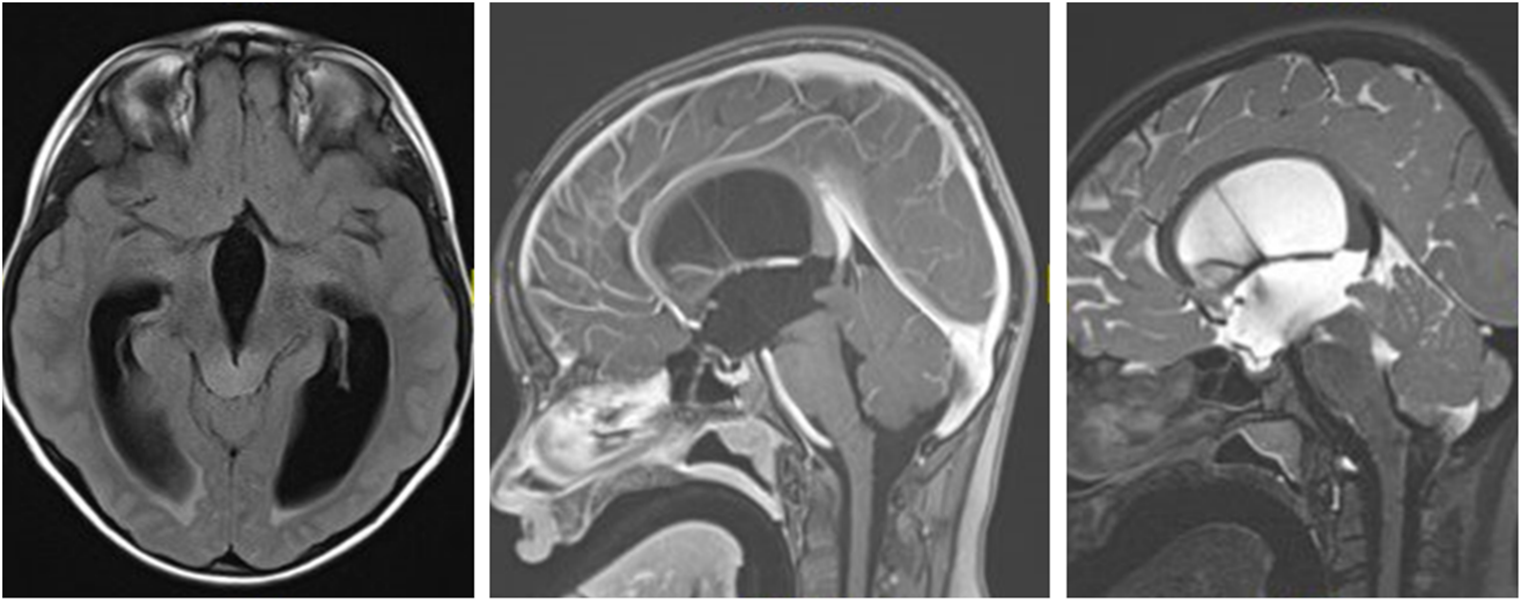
Tectal plate glioma (TPG): axial FLAIR, and sagittal T2-weighted and T1-contrast-enhanced MRI of a 12-year-old girl presenting with obstructive hydrocephalus. MRI shows an isointense lesion on T1-weighted imaging (middle image), without contrast uptake, slightly hyperintense on T2-weighted imaging (right image), leading to a “ballooned” tectum and compression of the aqueduct. The child underwent an ETV and is symptom-free for last 6 years, with no tumor progression
